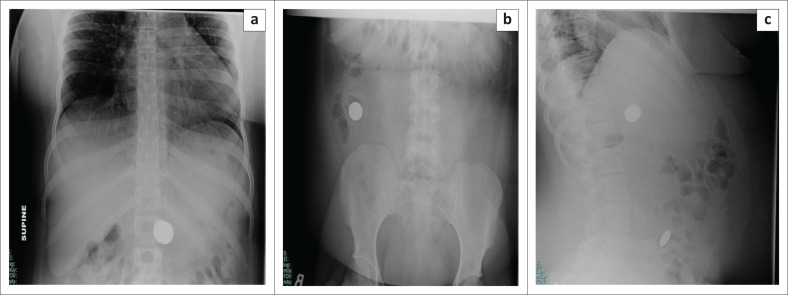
Serial abdominal radiographs frontal (a and b) and lateral (c) views of a 16-year-old female patient with a psychiatric history who presented with multiple coin ingestions and was managed conservatively.
